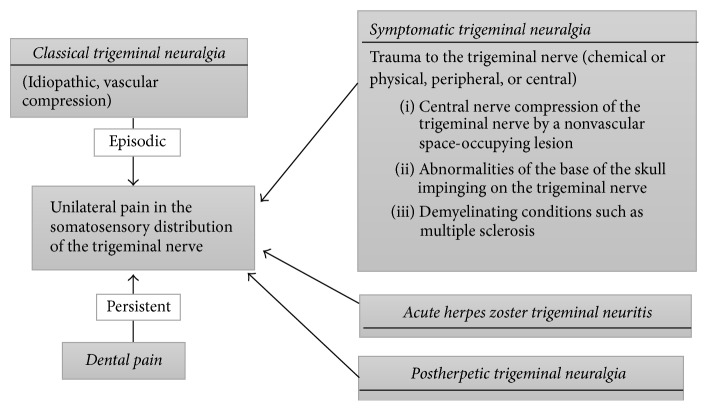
Orofacial neuralgic pain conditions that can occur in the somatosensory distribution of the trigeminal nerve. Careful examination, a thorough medical and dental history, and neuroimaging of the head with magnetic resonance imaging (MRI) are necessary for the diagnosis of classical and symptomatic trigeminal neuralgia [18, 24].
